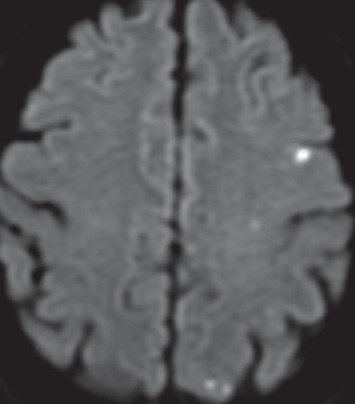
Diffusion MRI sequence showing embolic appearing regions of restricted diffusion (bright white spots) in the left hemisphere.
